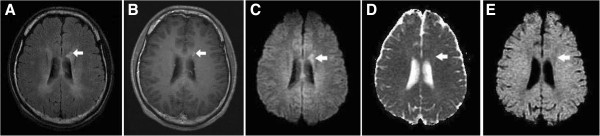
A type IB lesion in a patient with MS. (A) Axial T2-FLAIR brain MRI shows dirty appearance of the bilateral periventricular white matter and a demyelinating lesion on the left side (arrow). (B) The lesion (arrow) shows enhancement on the CE T1WI. (C–E) It is hyperintense on the DWI, isointense to slightly hyperintense on the ADC map and isointense to slightly hypointense on the eADC map. The appearances suggest an active stage of demyelination with conversion to vasogenic edema and the T2 shine-through effect.
